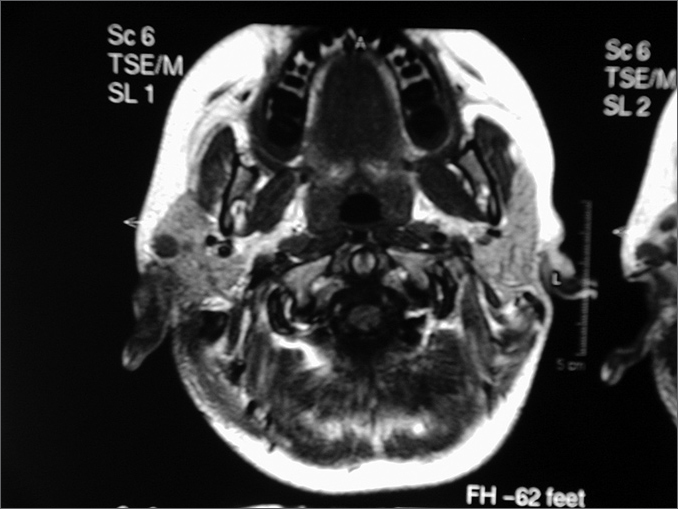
Magnetic resonance imaging of the cranium - presence of lesions in both temporal bones suggesting histiocytosis X.
